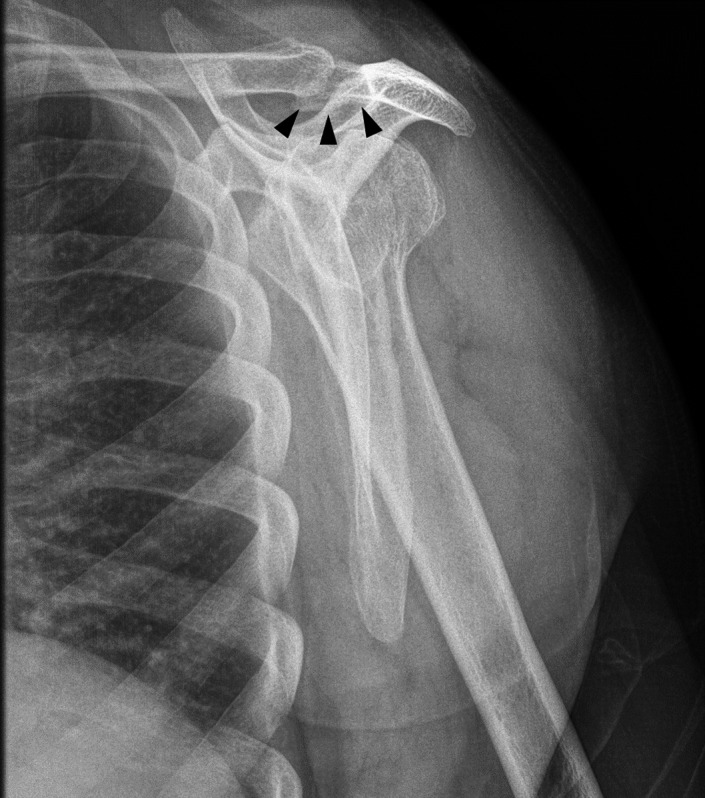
Scapular‐Y radiograph where a faint, extraskeletal, ossified area is depicted, underneath the acromioclavicular joint (black arrowheads)
